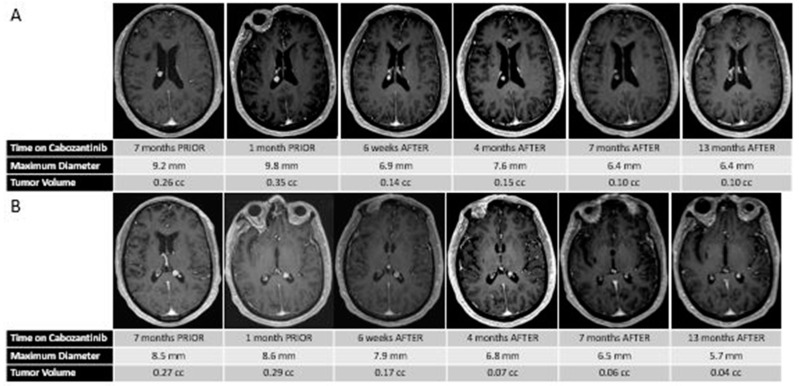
(A) Sequential MR images demonstrating the changes in size and volume of the right putative intraventricular meningioma before and after treatment with cabozantinib. (B) Sequential MR images demonstrating the changes in size and volume of the left putative intraventricular meningioma before and after treatment with cabozantinib.
